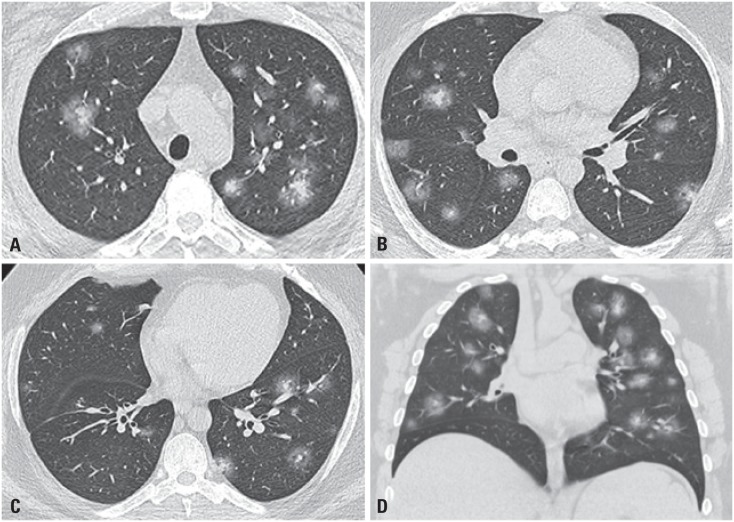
Halo sign. Axial (A and C) and coronal (D) images in multislice computed tomography show small multiple solid nodules with ground-glass halo in upper (A), middle (B), and lower (C) lung fields, representing the halo sign. Other nodular opacities with ground-glass attenuation can be seen
